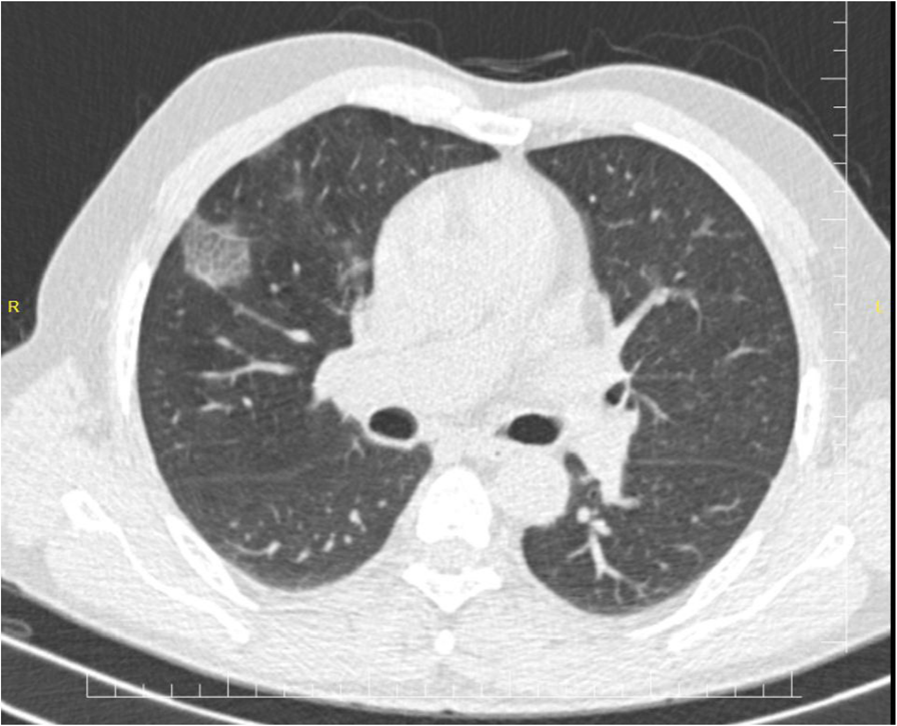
An axial chest CT from a 50-year-old man with COVİD-19 pneumonia. Non-contrast-enhanced chest CT image showed focal ground-glass opacity associated with smooth interlobular and intralobular septal thickening in the right upper lobe. The left lung was normal
